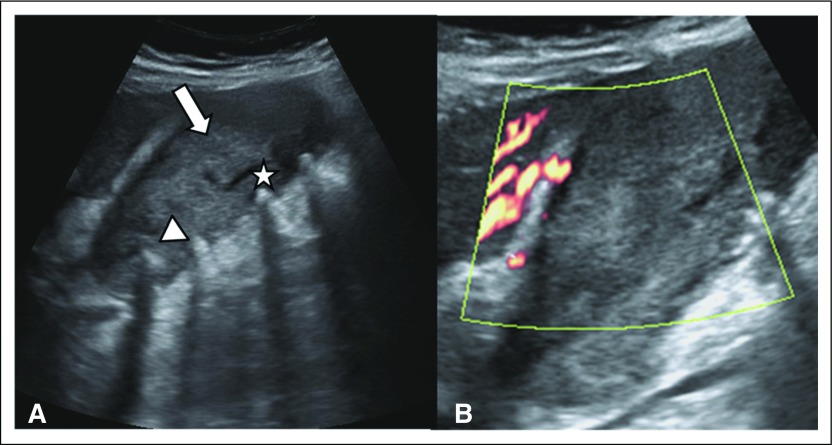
Ultrasonographic images of gallbladder in a 69-year-old man with right hypochondriac pain. (A) Longitudinal ultrasonographic image shows diffusely thickened gallbladder wall (arrow) with associated multiple intramural calculi (arrowhead) and echogenic intramural foci with comet-tail reverberation artifacts, indicative of cholesterol crystals within Rokitansky-Aschoff sinuses (star). (B) No obvious color uptake on power Doppler.
